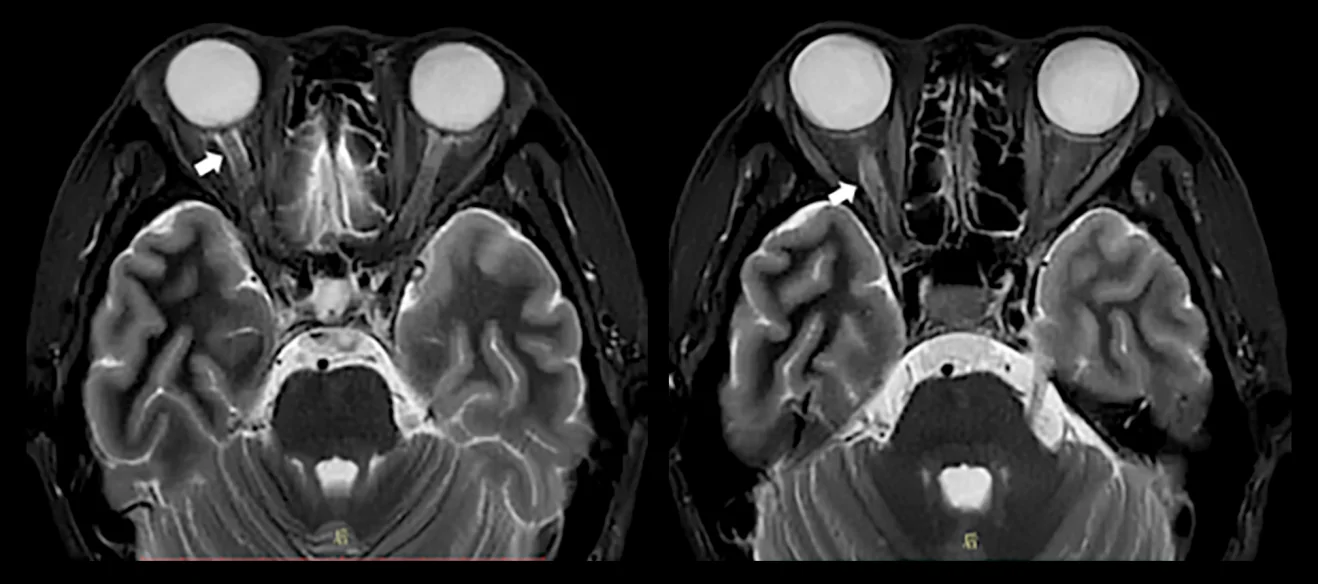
Neuroimaging hallmarks of post-infectious immune-mediated optic neuritis (MOGAD). Axial T2-weighted orbital magnetic resonance imaging (MRI) images from a 42-year-old woman with vision loss and seropositivity for MOG-IgG. The images (two views from the same sequence) demonstrate high-signal intensity involving the optic nerve sheath around the right optic nerve (white arrows), forming a typical “double track sign,” consistent with optic perineuritis reported in MOGAD-associated optic neuritis. This real-world example supports the role of neuroimaging in recognizing immune-mediated phenotypes in the appropriate clinical and serologic context. [Reprinted from (Wang MM. et al., 2024), under CC BY-NC-ND 4.0]. IgG, immunoglobulin G; MOG-IgG, myelin oligodendrocyte glycoprotein IgG; MOGAD, myelin oligodendrocyte glycoprotein antibody-associated disease; MRI, magnetic resonance imaging.
